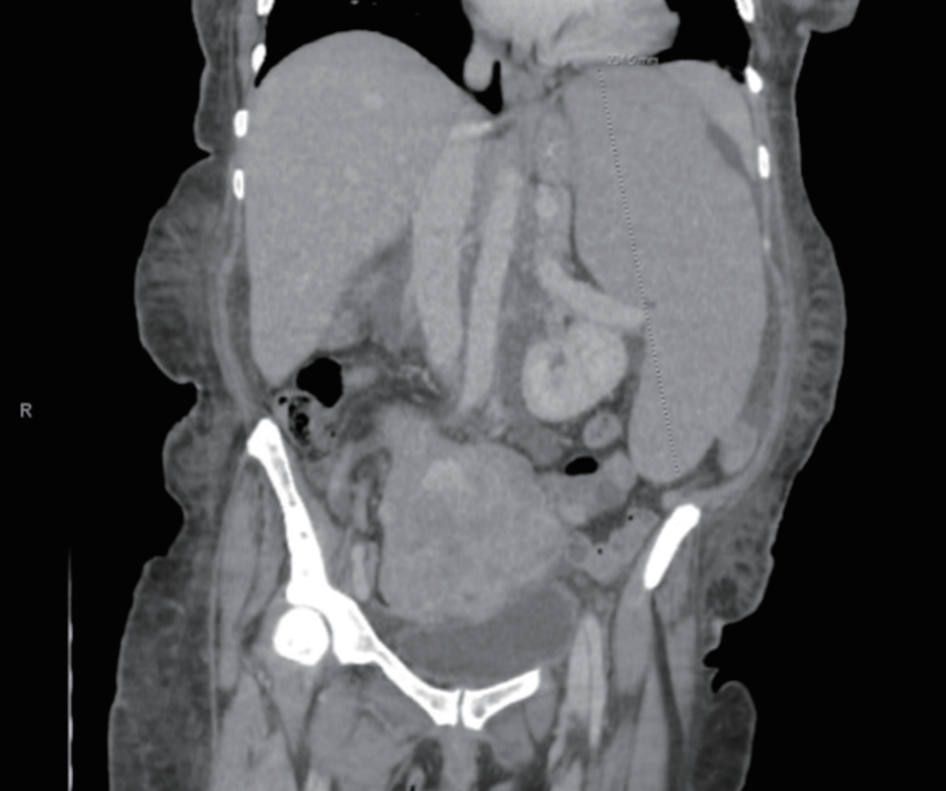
Coronal view of CT chest/abdomen/pelvis showing significant splenomegaly, measuring 23.5 cm in craniocaudal dimension. Enlarged, lobulated uterus with partially calcified and heterogenous fibroids are also seen. CT: computed tomography.
